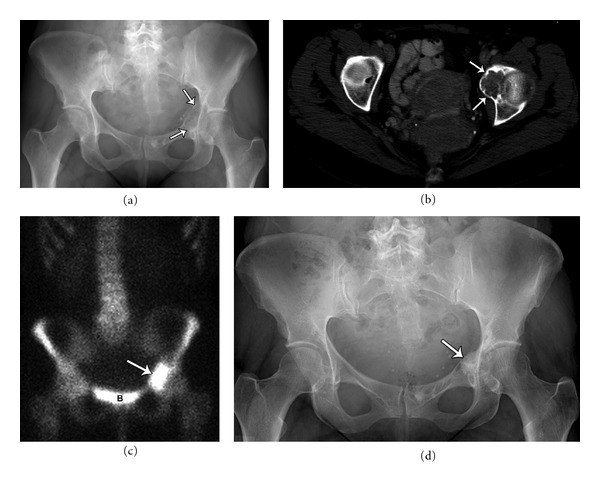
Forty-six-year-old female with a lytic lesion in the left medial acetabulum, diagnosed as chondroblastoma. Plain film (a) and axial CT (b) demonstrating a lytic lesion with heterogeneous chondroid matrix and sharp scalloped margins. The tumor showed increased uptake on bone scan (c) (B: bladder). Plain film (d) demonstrating healed chondroblastoma following surgical curettage and cement packing.
